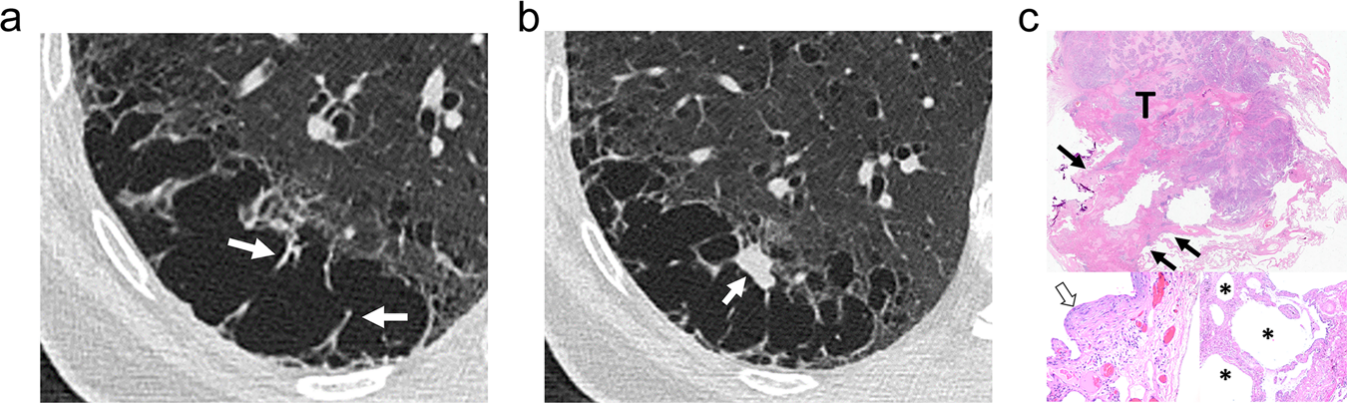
Traction emphysema lesions in a 62-year-old male smoker who underwent a right lower lobe lobectomy for lung cancer. a CT shows irregular cystic lesions extending along the pleural surface exhibiting interrupted septa in their walls, described as the “stalactite and stalagmite sign” (arrows). b A lung nodule (arrow) is seen in the wall of this traction emphysema cyst. At pathology (c), dense collagen fibrosis (black arrows) next to the tumoral lesion (T) is seen. Away from these lesions, there were findings of a UIP pattern with fibroblastic foci (open arrow) and honeycombing cysts (asterisks)
